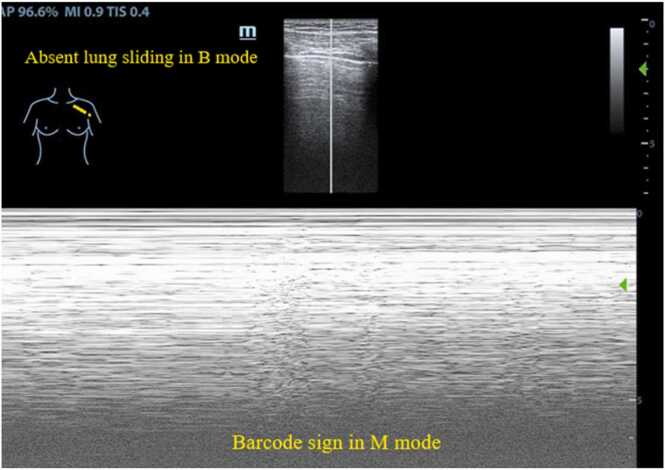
Representative of ultrasound image findings for obstructive shock; Absent lung sliding in B mode and Barcode sign in M mode.
